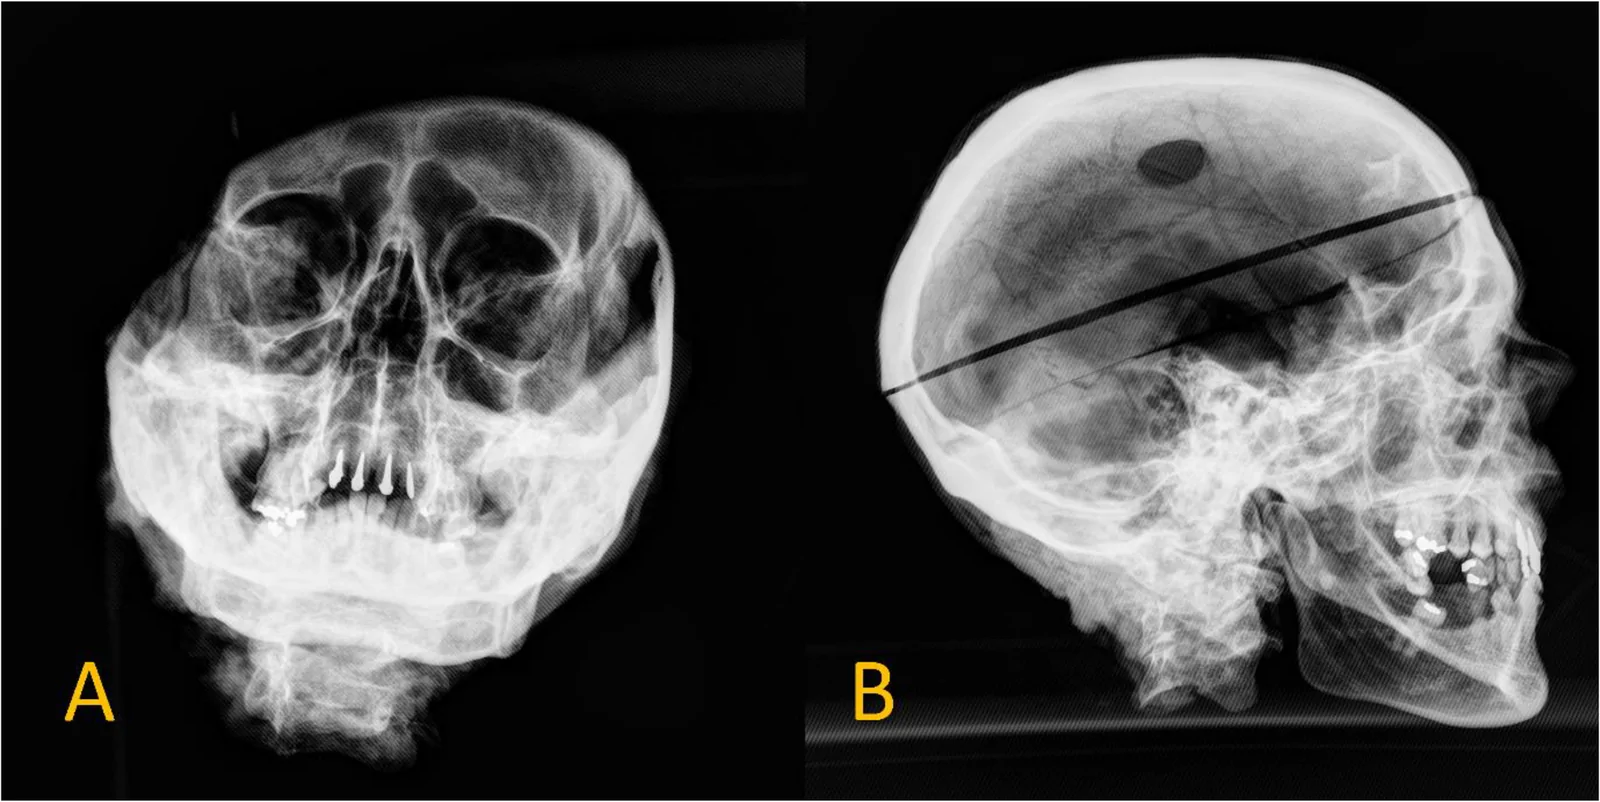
Radiographs of the paranasal sinuses: (A) frontal view and (B) lateral view
